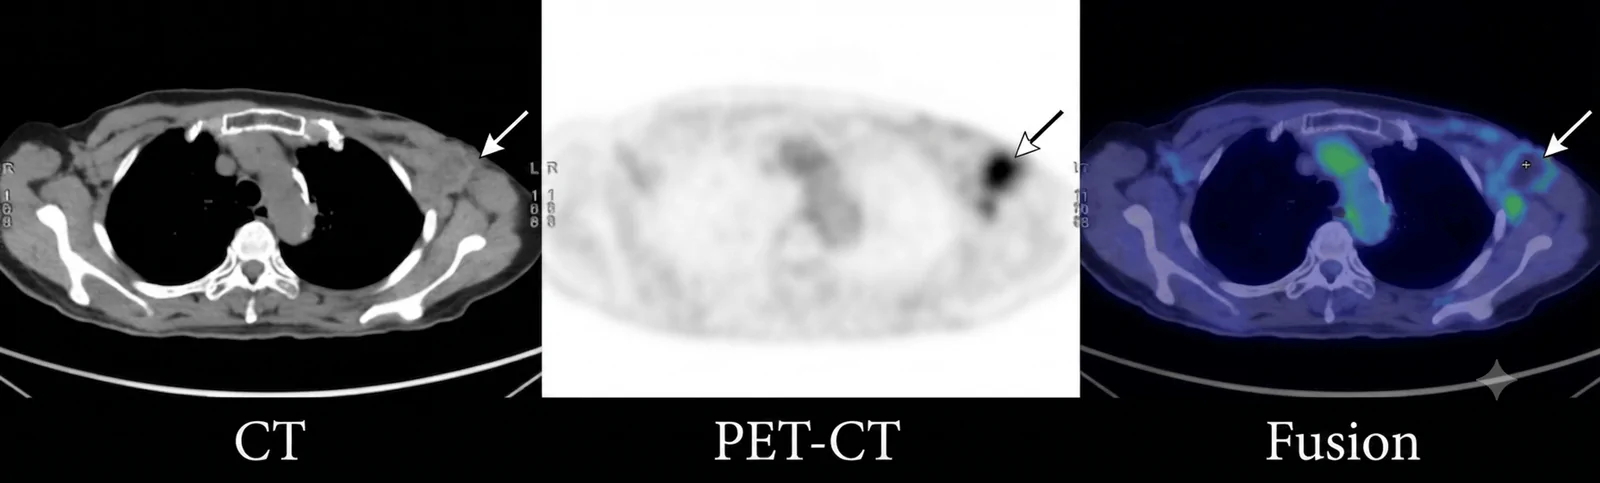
Axial CT, PET, and fused PET-CT images demonstrating the left axillary/chest wall lesion. Axial cross-sectional imaging of the left axillary/chest wall region displaying the lesion via noncontrast CT, PET, and fused PET-CT modalities. White arrows indicate the location of the axillary mass, highlighting its anatomical and metabolic characteristics. CT, computed tomography; PET, positron emission tomography
